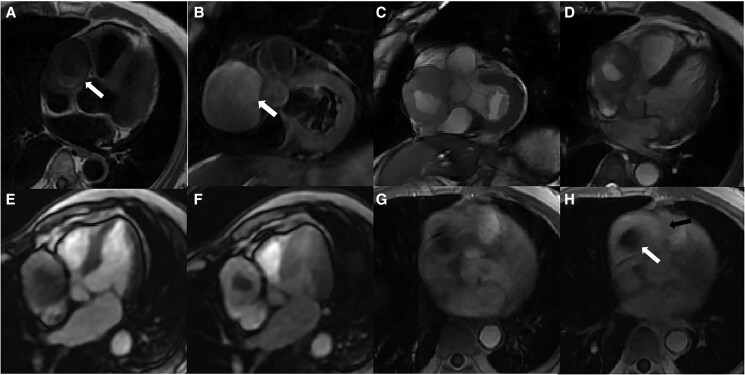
Cardiac magnetic resonance showing a mixed cystic and solid tumour compressing the right atrioventricular sulcus area. (*A*, *B*) The centre of the tumour presented low signal intensity, while the peripheral region shows a ring isointensity in axial T1-weighted imaging (*A*) (white arrow), but no signal reduction was observed in T2-weighted imaging fat suppression sequence (*B*) (white arrow). (*C*, *D*) The short-axis and four-chamber cine views showed T2-hyperintense the location of the tumour. (*E*, *F*) The solid part of the tumour was gradually enhanced in rest first-pass perfusion. (*G*, *H*) Early and late enhancement showed ring uniform enhancement of the solid components (white arrow), and no enhancement was observed in the central area of the tumour (black arrow).
